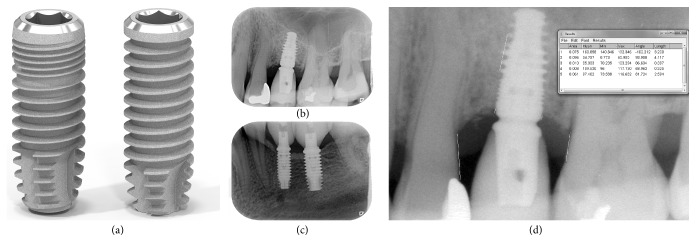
Representative test ((a), left: SICmax, SIC invent AG, Basel, Switzerland) and control implant ((a), right: SICace) of comparable dimensions (platform: 4.2/4 mm, length: 11.5 mm) to be installed in identical implant bed dimensions and exemplary standardized radiographs of a test implant installed in the maxilla (b) and two adjacent control implants installed in the mandible (c) at the 1-year follow-up. (c) shows representative measurements of MBL at the mesial and distal aspect of a test implant with the image analysis software (ImageJ) to be compared with the baseline values gathered at implant installation.
